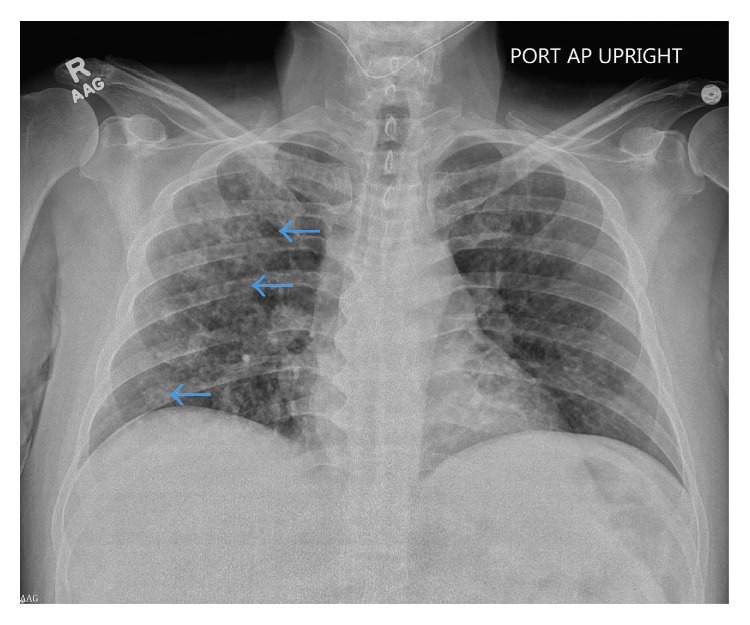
Chest roentgenogram on admission to our hospital. AP chest radiograph at presentation. Again seen are diffuse micronodules with confluent areas in the right upper and lower lungs. Hint of small cavities is again noted (blue arrows).
